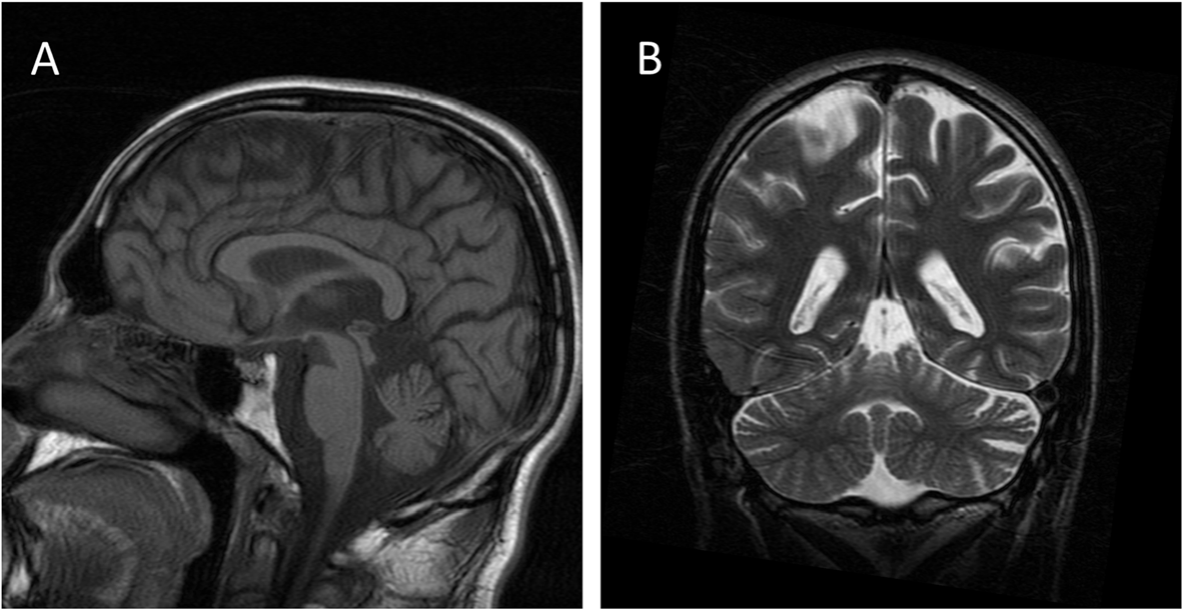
Brain MRI T1 (A) and T2 (B) sequences showing atrophy of vermis and cerebellar hemispheres
